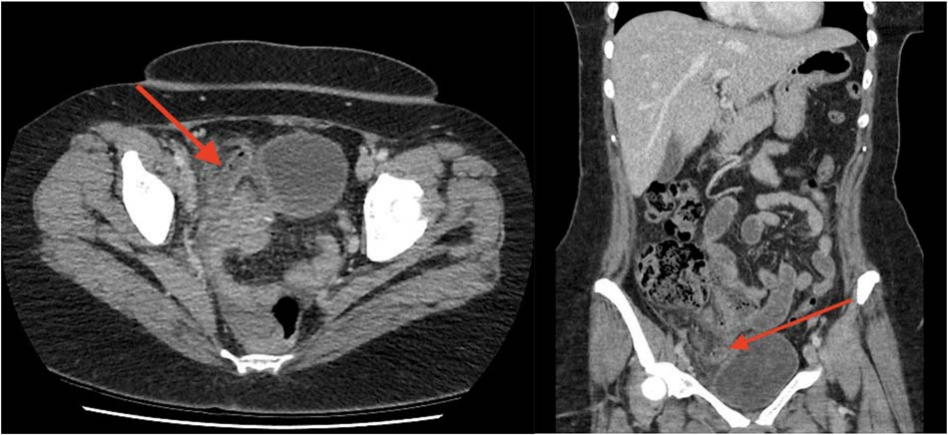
Axial (a) and coronal (b) images from portal-venous phase CT of the abdomen and pelvis demonstrating acute appendicitis. Arrow to inflamed, enlarged appendix.
